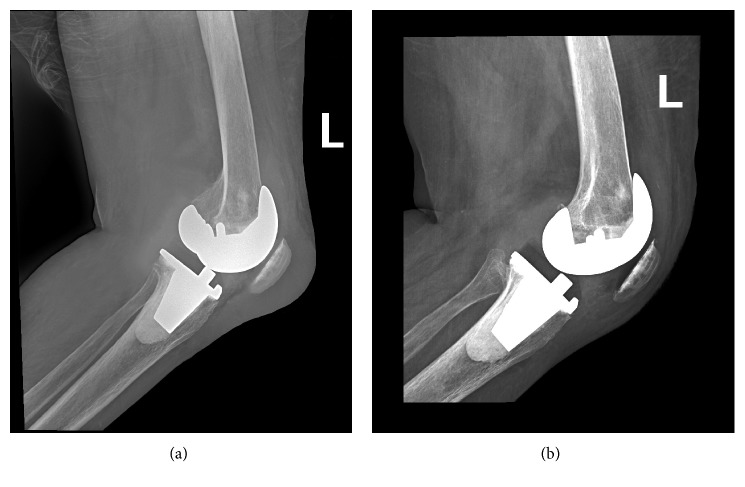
(a) Plain lateral view radiograph of the left knee showing the prepatellar soft tissue swelling and otherwise well-fixed prosthesis with no signs of osteolysis at the bone-prosthesis interface or at the patella. (b) Plain lateral view radiograph of the same knee at the 6-year follow-up showing regression of the swelling and otherwise normal appearance of the prosthetic knee and the patella.
